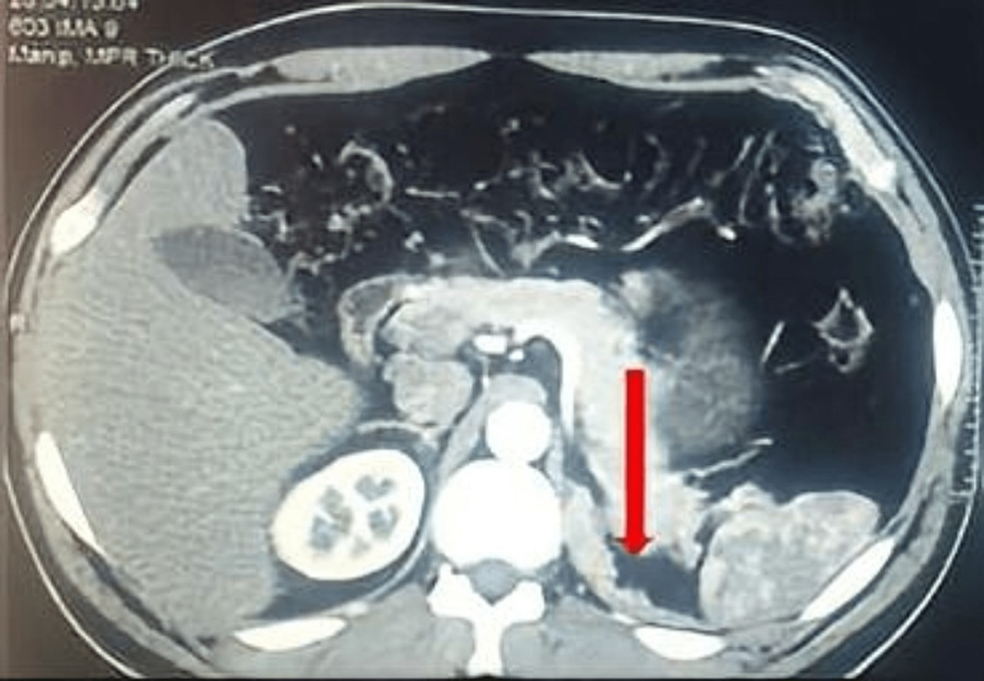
Multiphasic CT of the adrenals transverse view, showing a new irregular tumour growth at the site of the previous tumour excision (arrow).
